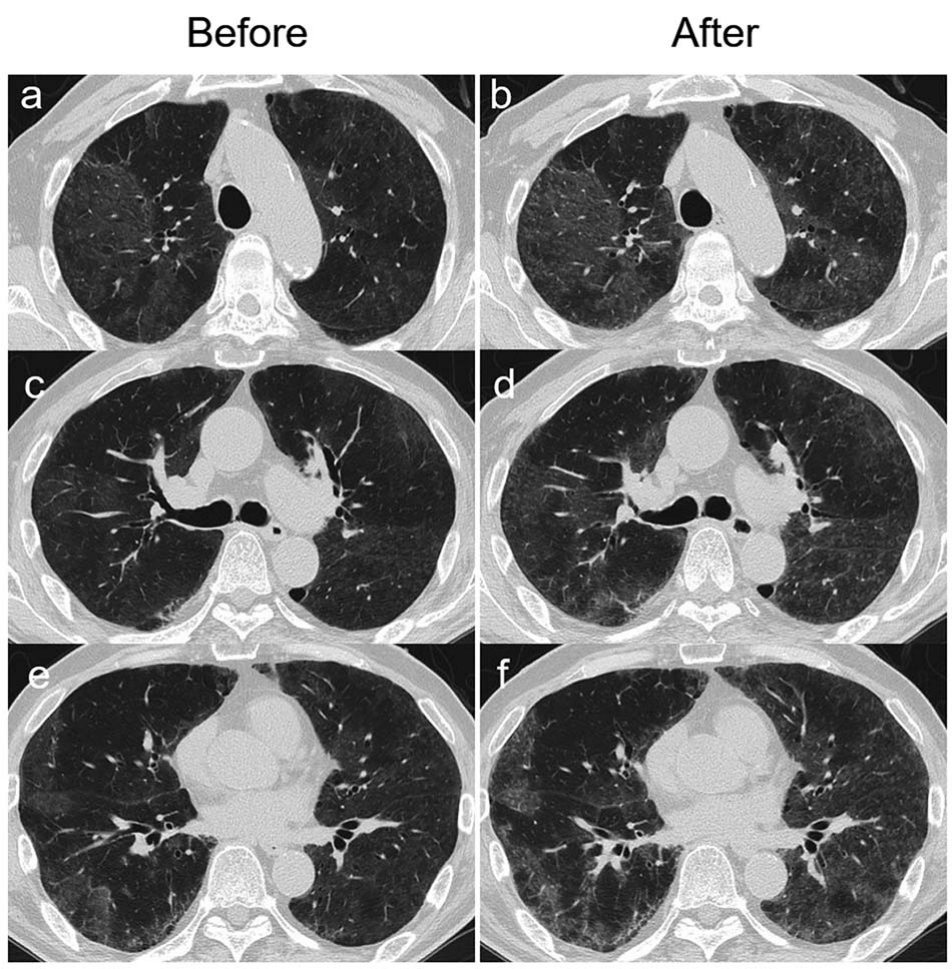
Chest HRCT images taken before and 9 h after the inhalation challenge test (ICT) with humidifier water performed on hospitalization Day 9. The HRCT scan taken before the ICT (a, c and e) shows the improvement of GGOs compared to the HRCT scan taken on the hospitalization Day 1 (Fig. 1b, d and f). After the ICT, GGOs worsened again (b, d and f).
